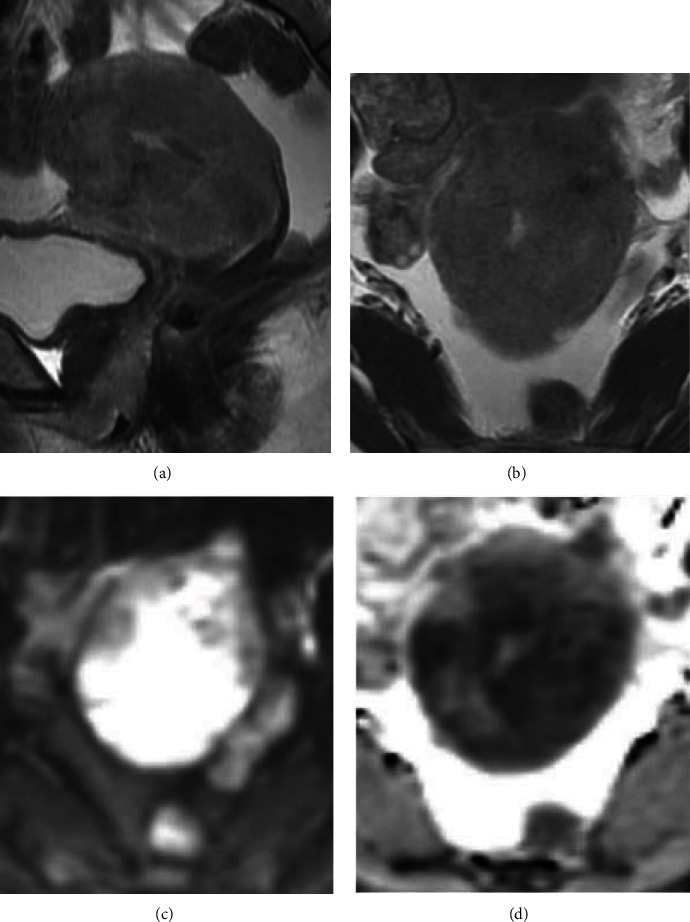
(a) Sagittal T2-weighted MRI image of the uterus and the vagina: the myometrium signal intensity was higher than that of the intestinal stroma. Similar intensity was observed in the lower vagina, the vulva, and a nodule on Douglas' pouch. (b) Axial T2-weighted MRI image of the uterus and the pelvis. The signal intensity of the myometrium was higher than that of the coccygeus muscle. (c) Axial diffusion-weighed image of the uterine body. The entire myometrium showed high intensity. (d) Apparent diffusion coefficient (ADC) map. The mean ADC value of the whole uterus was 0.950 × 10^−3^ mm^2^/s with 0.720 × 10^−3^ mm^2^/s of it being covered by the darkest shade.
